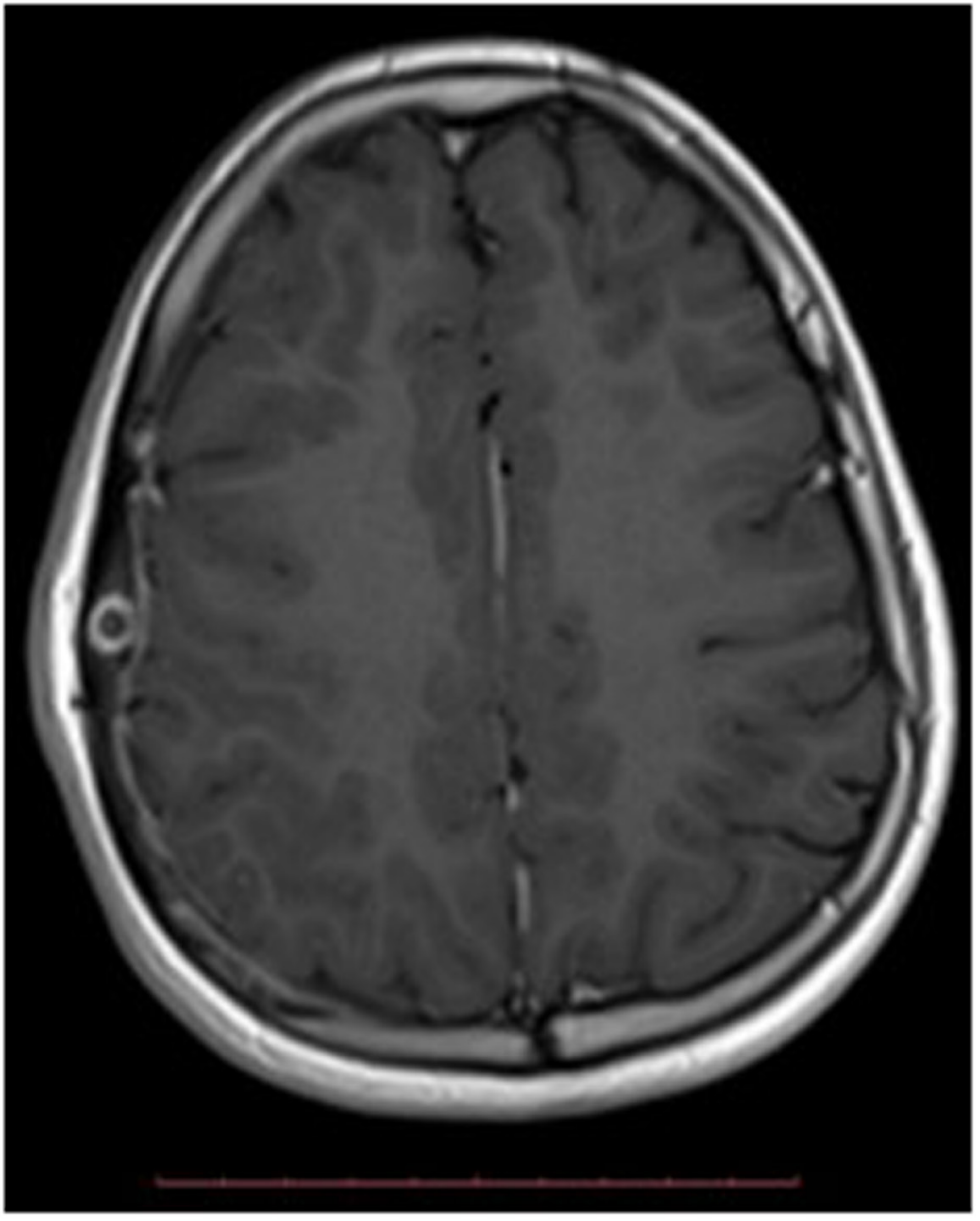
Axial T1‐weighted plus contrast MR image shows ring enhancement and also adjacent pachymeningeal enhancement
